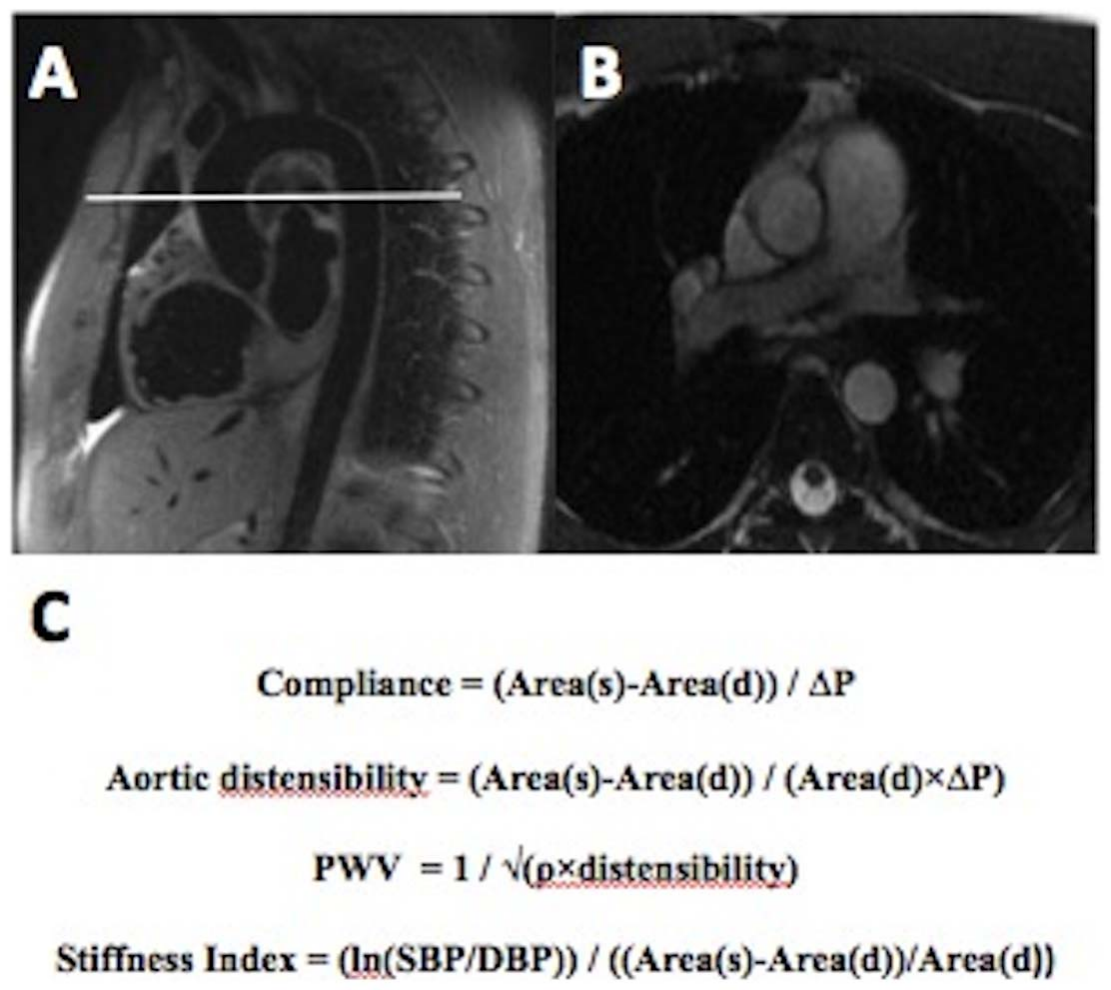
A–B. Scout cardiovascular magnetic resonance image of thoracic aorta demonstrating the planning of a transverse section through proximal descending aorta at the level of the right pulmonary artery. C. Arterial stiffness equations. Area(s) = systolic area, area(d) = diastolic area, ΔP = SBP-DBP, ρ = blood density (1059 kg.m^−3^).
